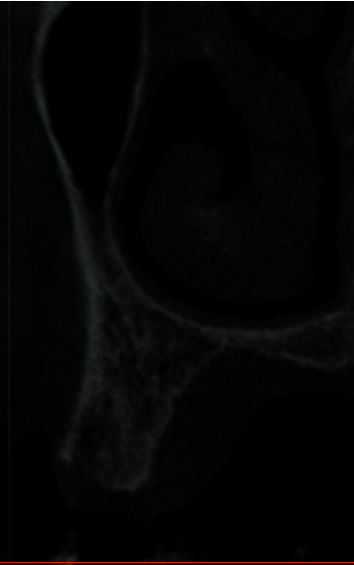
CBCT image before implant placement.
